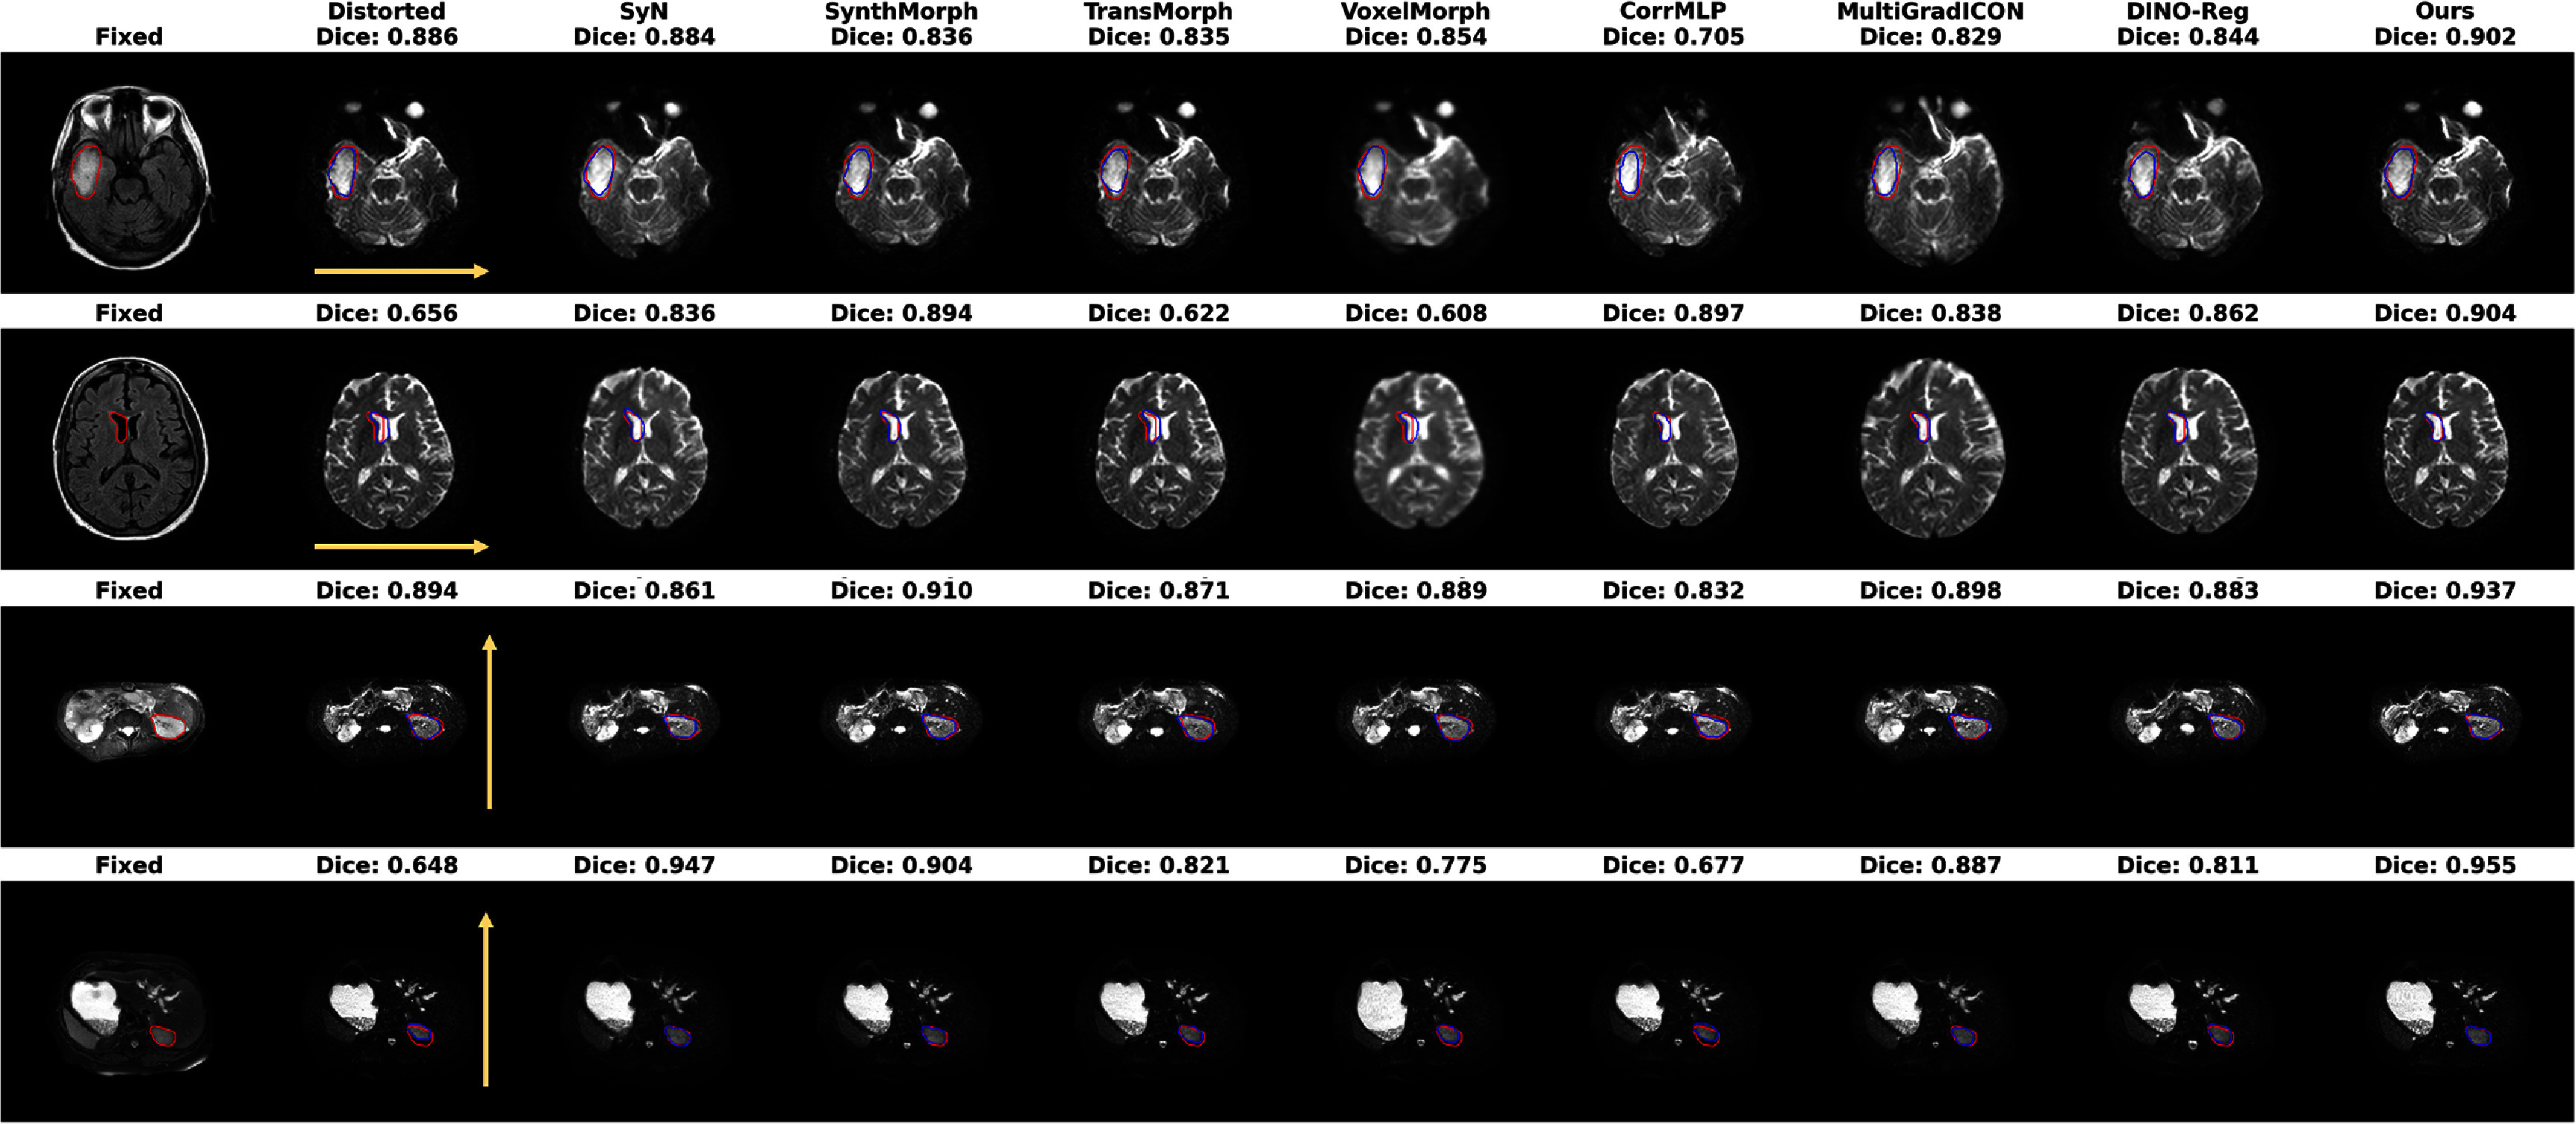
ROI-based evaluation on the clinical DWI data. Upper two rows show brain cases and lower two rows show abdominal cases. Red contours represent ROIs delineated on the fixed anatomical references (FLAIR for brain, and T2-SPIR for abdomen), while blue contours show corresponding ROIs on the registered DWI images from different methods. Yellow arrows indicate the phase encoding direction. Dice coefficients quantify the overlap between red and blue ROIs, with higher values indicating better geometric distortion correction. The proposed method achieves superior anatomical alignment across all evaluated structures.
